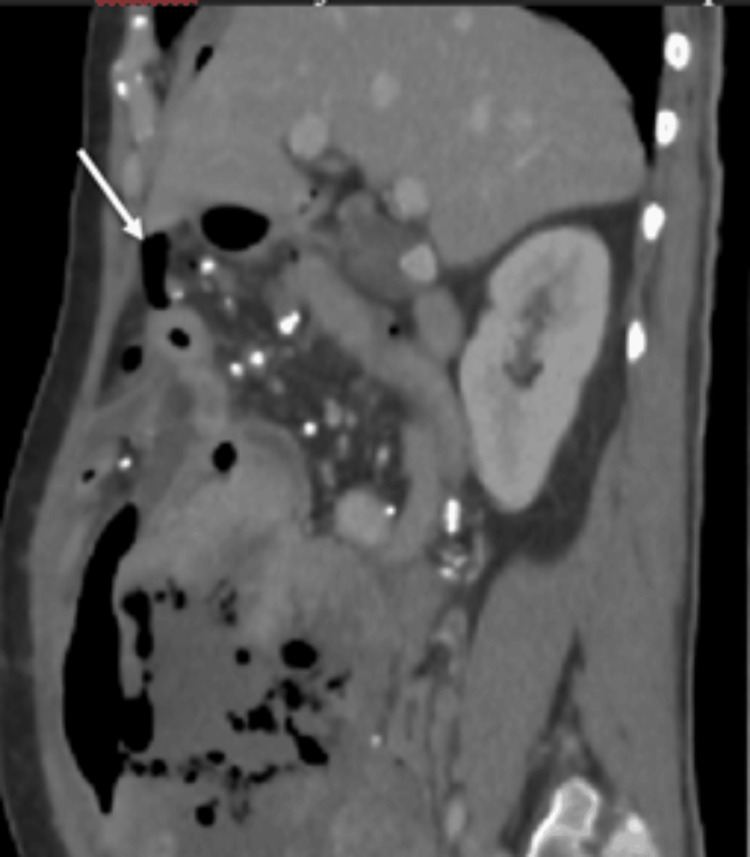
Sagittal CECT image in venous phase demonstrating pneumoperitoneum with air tracking from the tumor into the peritoneal cavity (white arrow) CECT: contrast-enhanced computed tomography
